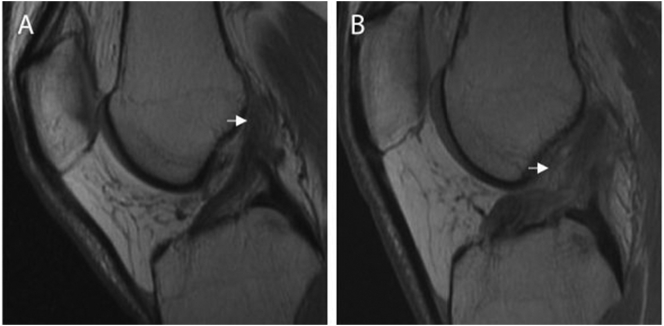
MRI of the same patient with bilateral ACL injuries. (A) Sagital T1 imaging of the right knee shows a proximal avulsion type 1 complete ACL tear (arrowhead). (B) Sagital magnetic resonance image T1 of the left knee shows a proximal type 2 complex complete ACL tear (arrowhead).
